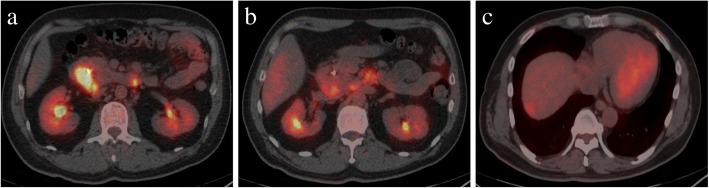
a Fluorodeoxyglucose positron emission tomography (FDG-PET) examination showed abnormal uptake in the tumor of the duodenum. b FDG-PET examination showed abnormal uptake in the para-aortic lymph nodes. c FDG-PET examination showed abnormal uptake in segment 8 of the liver
